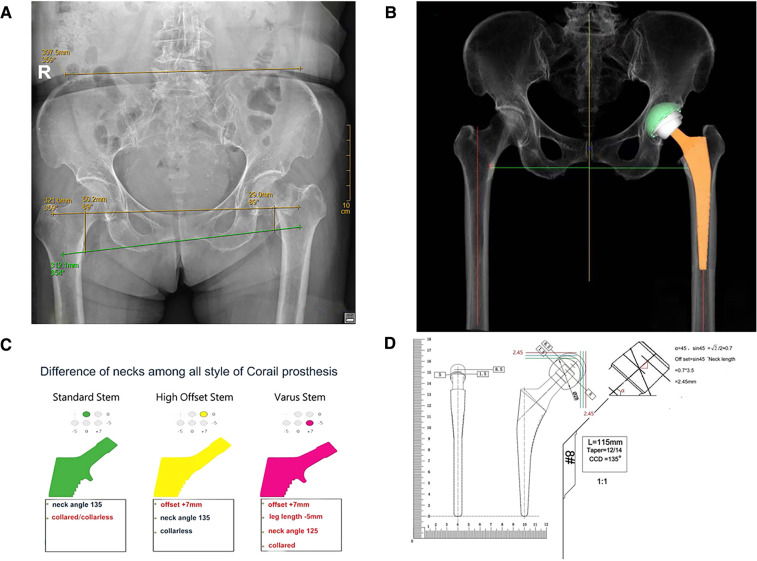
(A) Preoperative orthoptic pelvic x-ray measurement: the line connecting the lower edge of the Köhler teardrops on both sides was used as the pelvic reference line (yellow line), and the vertical distance from the apex of the inner edge of the lesser trochanter to the line connecting the Köhler teardrops on both sides represented the lengths of the lower limbs on both sides (red line); (B) the AIHIP software; (C) final state after planning by the AIHIP system. (D) The actual deviation among same size ball head (short, standard and long) horizontal and vertical.
